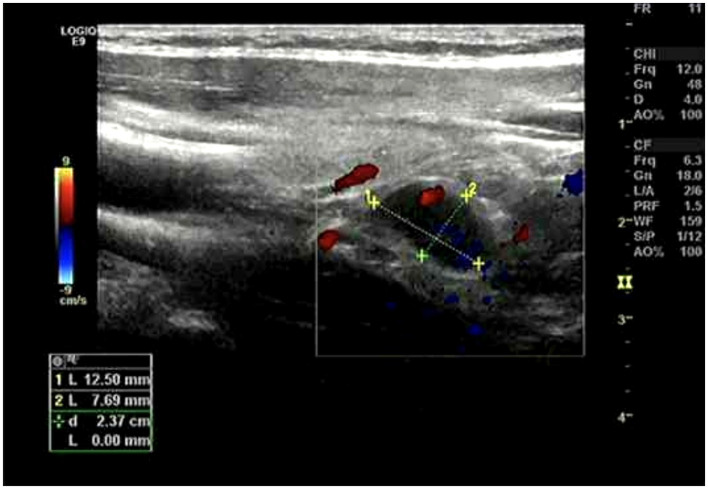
Ultrasound examination demonstrated a hypoechoic nodule located posterior–inferior to the right thyroid lobe, suggestive of enlarged and functionally active parathyroid tissue.
